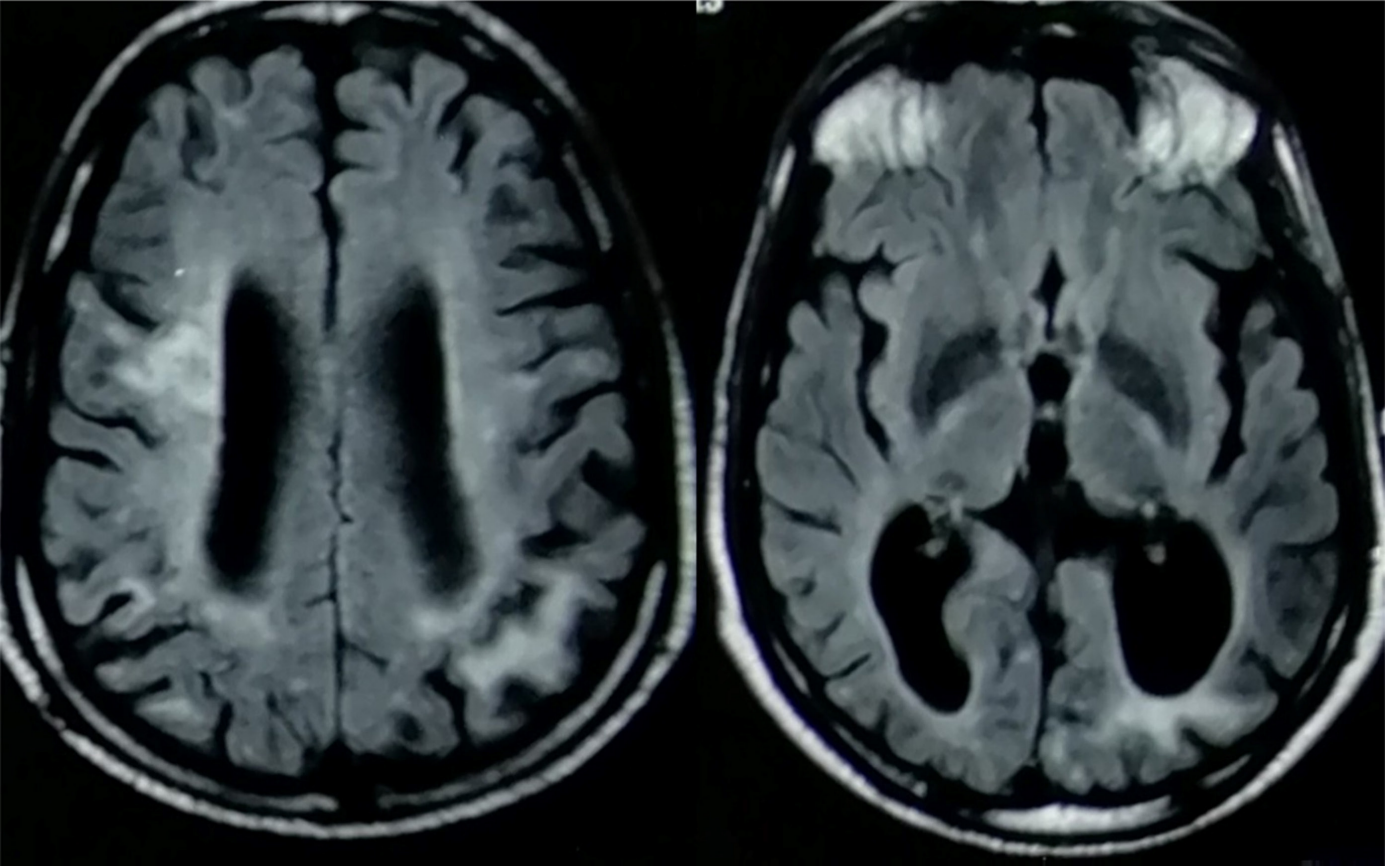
Brain MRI showed multiple ischemic lesions on T2‐weighted, some Gadolinium‐enhanced cortical–subcortical regions and on the left occipital lobe and left and right parietal lobes, and diffuse brain atrophy.
